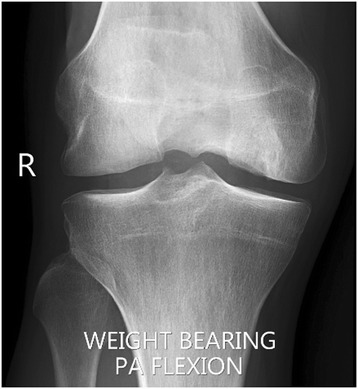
X-ray of the knee showing flattening of the medial femoral condyle and intercondylar notch osteophyte formation – Kellgren Lawrence Grade II
